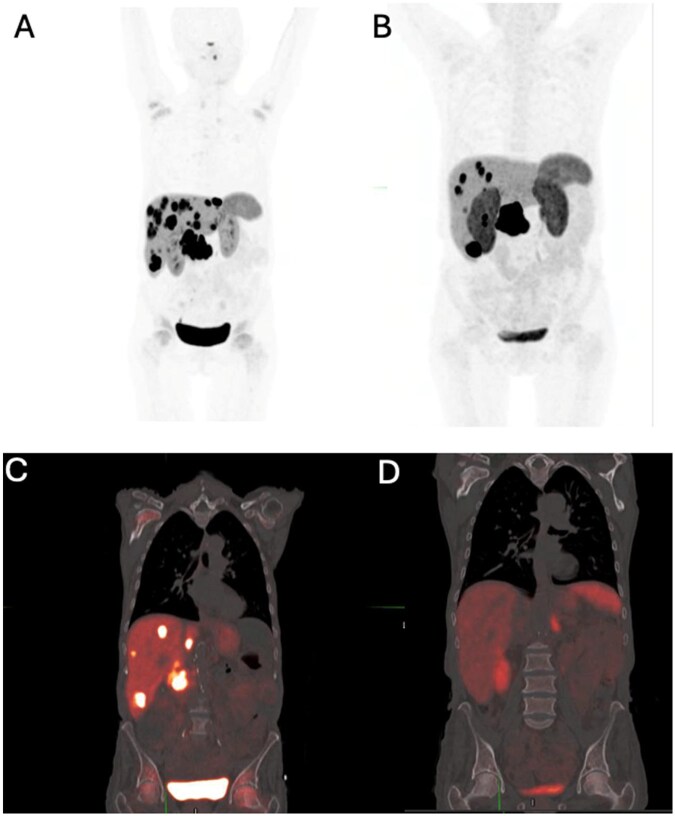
Radiographic response to pembrolizumab on ^64^Cu-DOTATATE PET/CT imaging. Maximum intensity projection (MIP) images (A, B) and corresponding fused PET/CT images (C, D) are shown. Baseline imaging obtained in October 2025 (A, C) demonstrates extensive somatostatin receptor-avid metastatic disease involving the liver and abdominal cavity. Follow-up imaging after four cycles of pembrolizumab in March 2026 (B, D) shows a marked reduction in radiotracer uptake and overall tumor burden, consistent with a significant treatment response.
